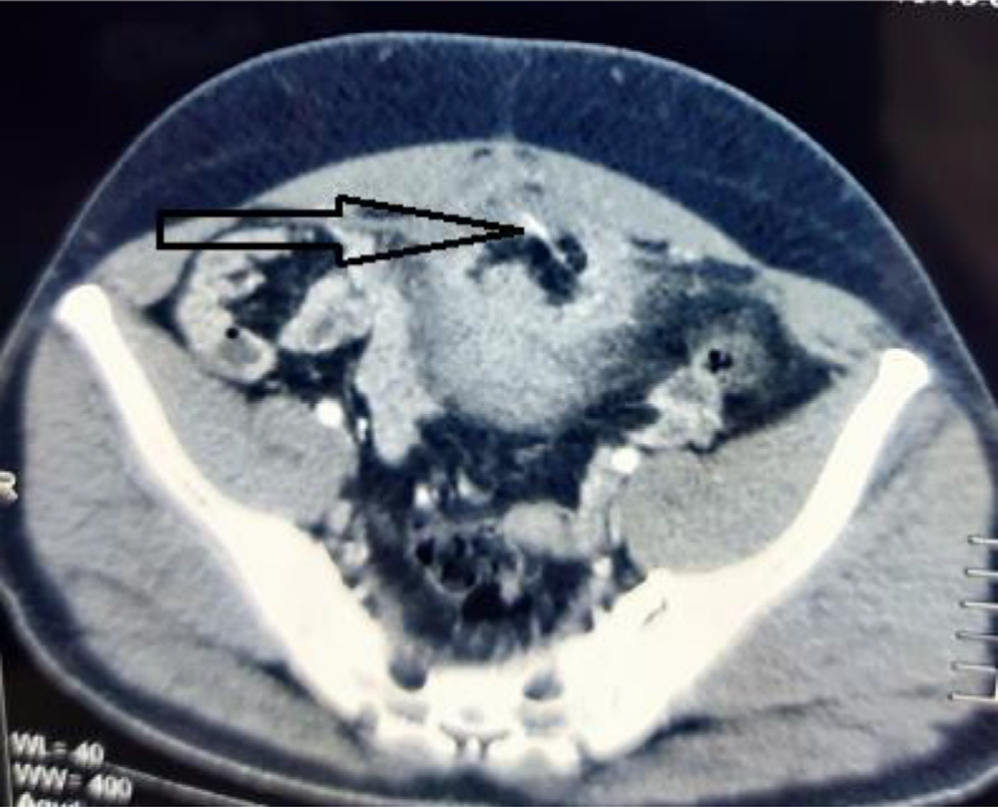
Axial CT image showing mass with linear calcified streak and central necrosis (white arrow).
